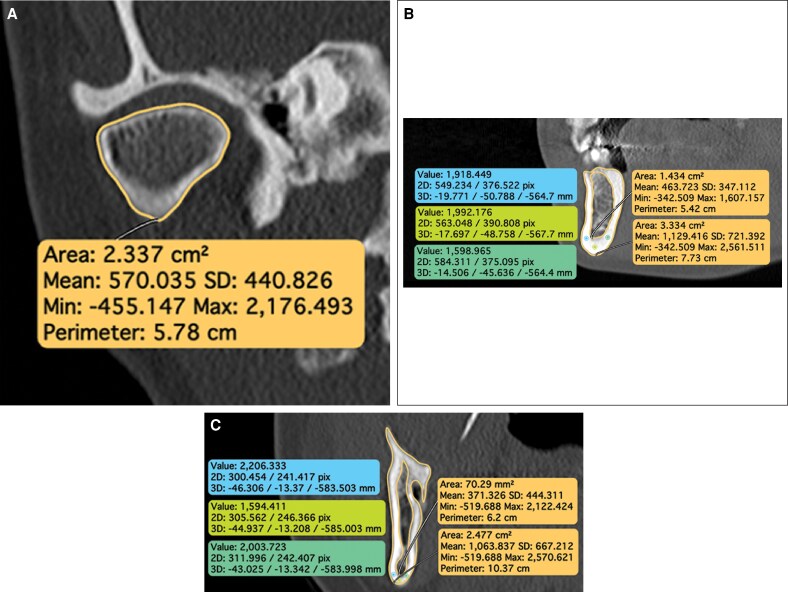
Example of (A) condyle, (B) premolar/alveolar, and (C) ramus measures. A single CT slice (1 or 3 mm, as appropriate) was employed for evaluations of all 3 regions of interest.
